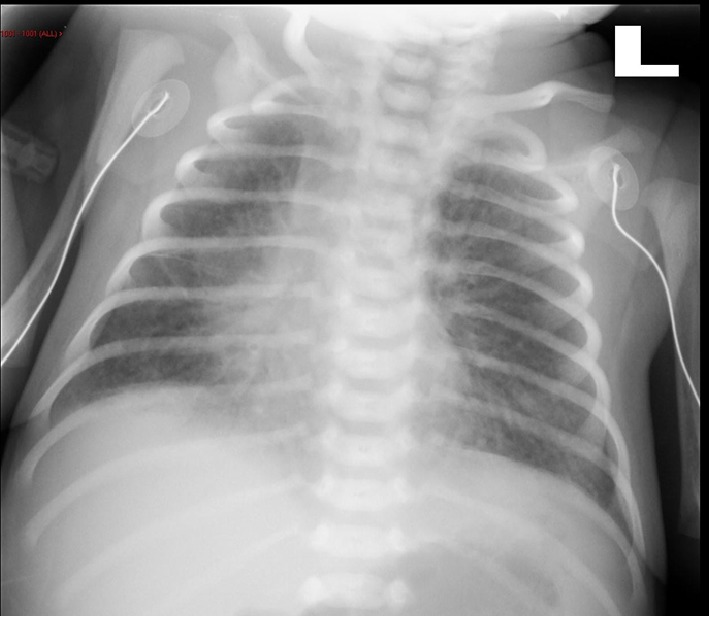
Chest X-ray on day 4. Diffuse interstitial pattern with normal lung volume.
